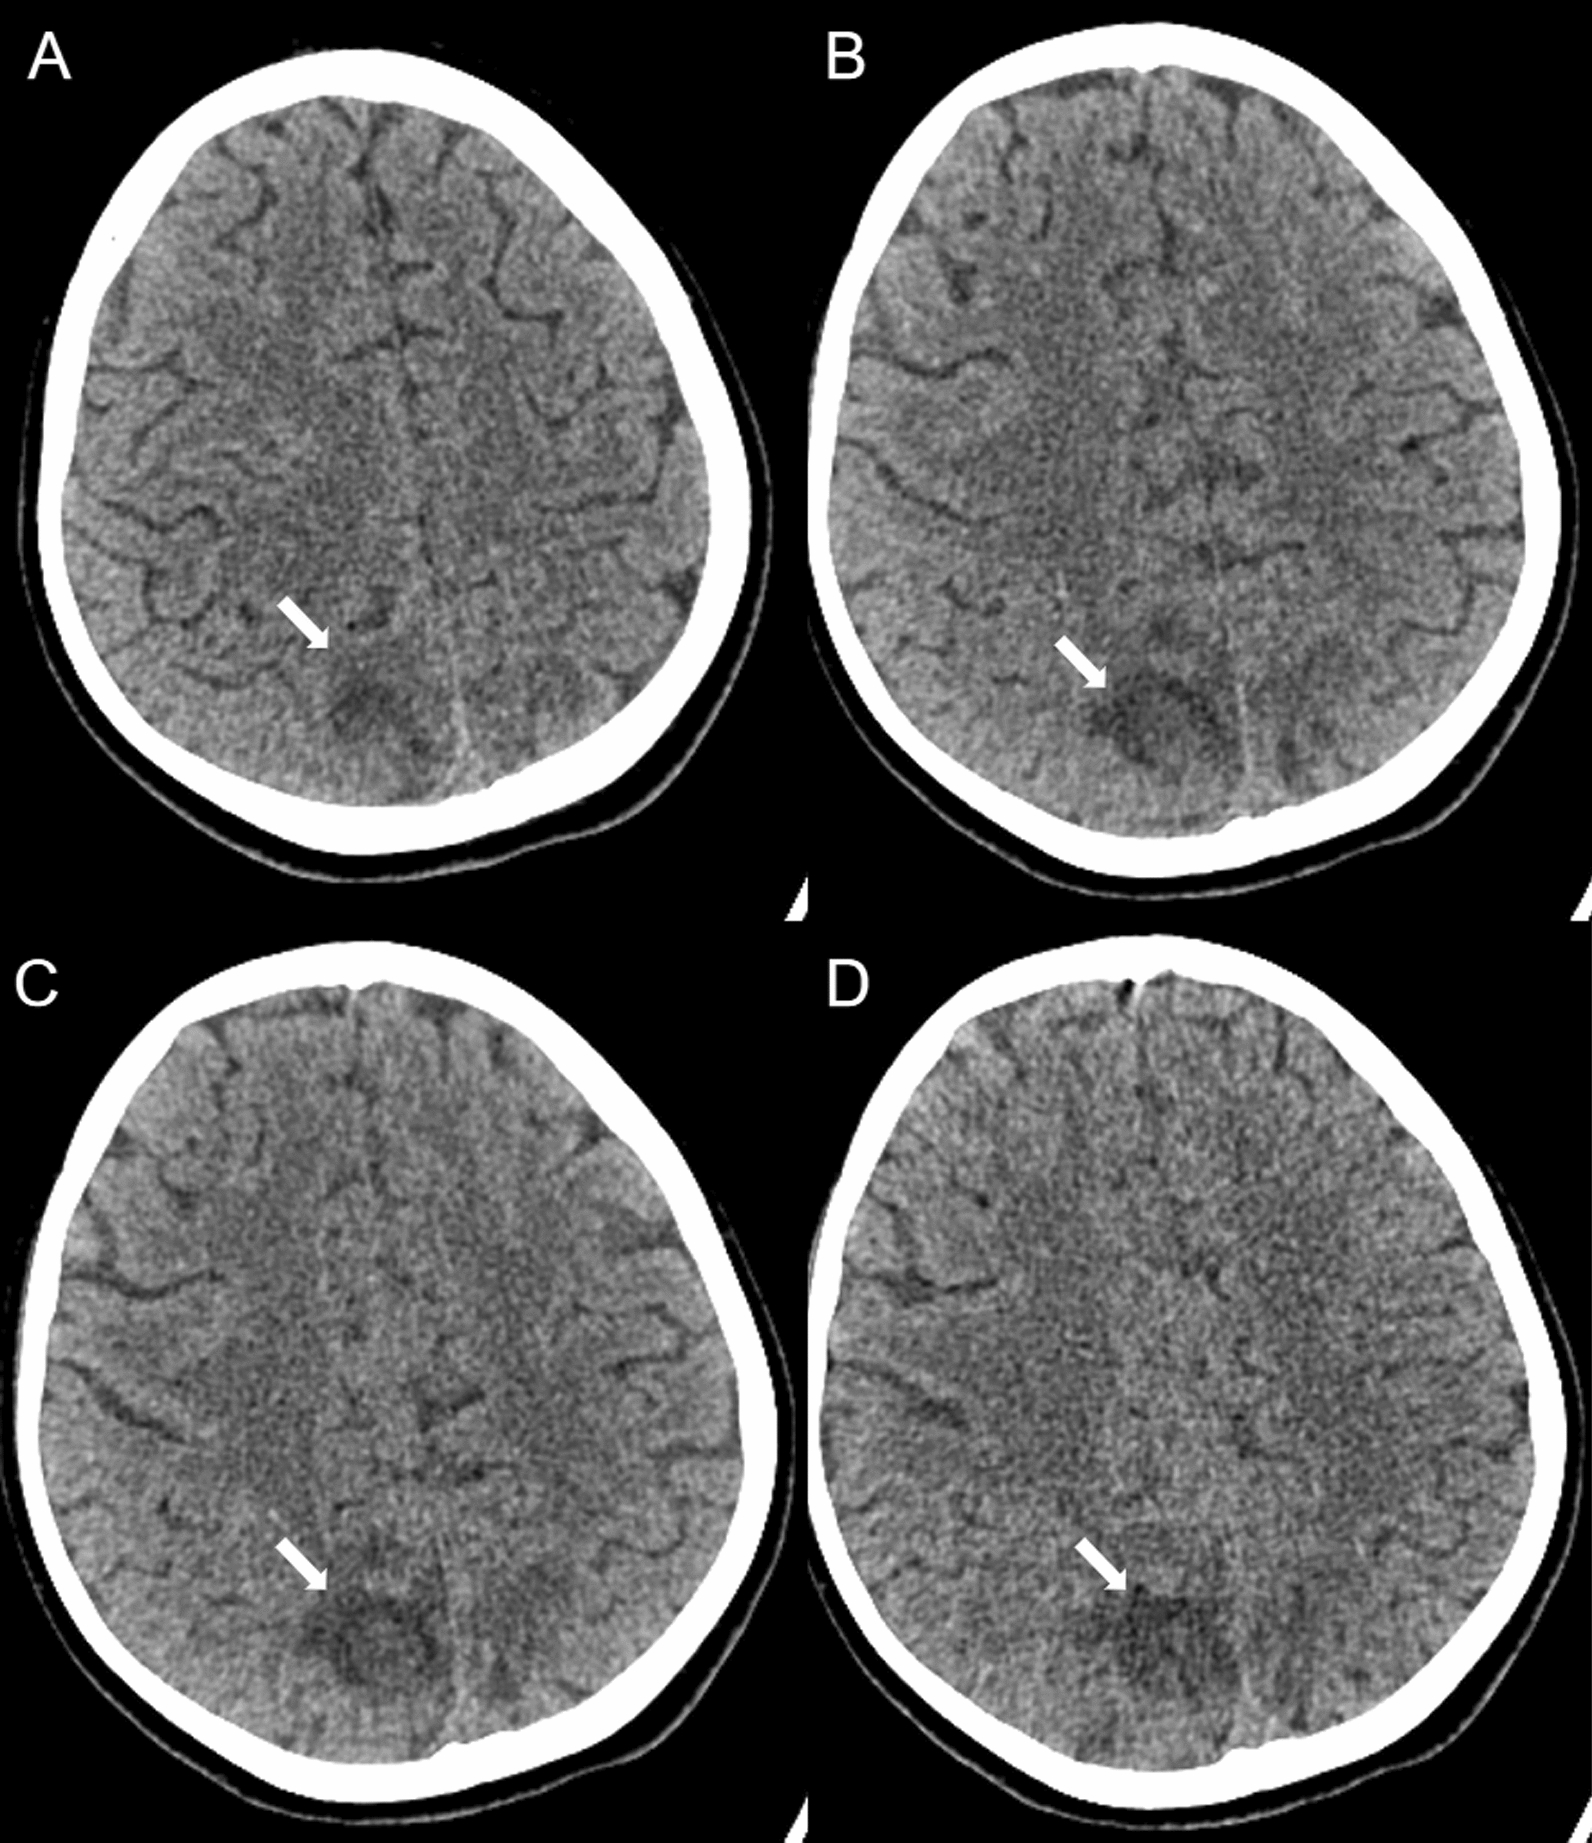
The CT manifestation of PRES, A-D :Bilateral Pariedtal-Occipital lobe cortex and subcortical patchy low-density shadows(the white arrow)
